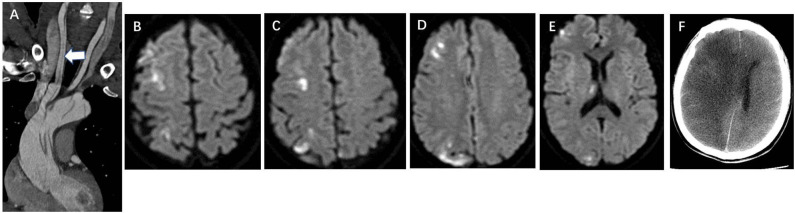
Cerebral infarction lesions distributed ≥3 lobes in the unilateral hemisphere is a DWI risk finding for postoperative new stroke in a 45-year-old man with ATAAD. (A) CTA oblique coronal MPR image shows aAO and aortic arch dissection involving whole right CCA (white arrow). (B–E) Preoperative diffusion-weighted magnetic resonance image shows multiple high signal of cerebral infarctions distributed in the frontal lobe, parietal lobe, occipital lobe, and thalamus in different layers of the right hemisphere. (F) Brain CT 20 days after surgery shows a massive cerebral infarction in the right hemisphere. DWI, diffusion-weighted magnetic resonance imaging; ATAAD, acute type A aortic dissection; CTA, computed tomography angiography; MPR, multiplanar reformation; aAO, ascending aorta; CCA, common carotid artery.
